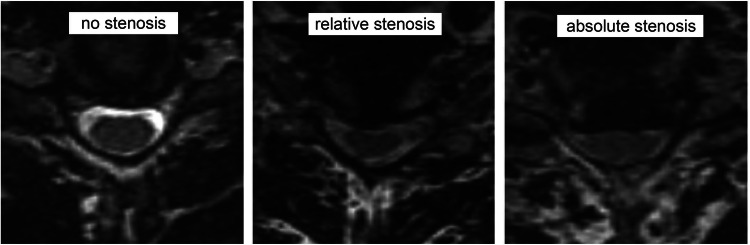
Subjective categorization of cervical spinal stenosis. Exemplary transverse T2-weighted images at level C5/6 for all three subjective stenosis categories: “no stenosis” = no degenerative elements contacting the spinal cord; “relative stenosis” = focal narrowing of the CSF space with contact to the spinal cord or circumferential narrowing with residual CSF signaling; “absolute stenosis” = absent CSF space with or without spinal cord volume reduction.
